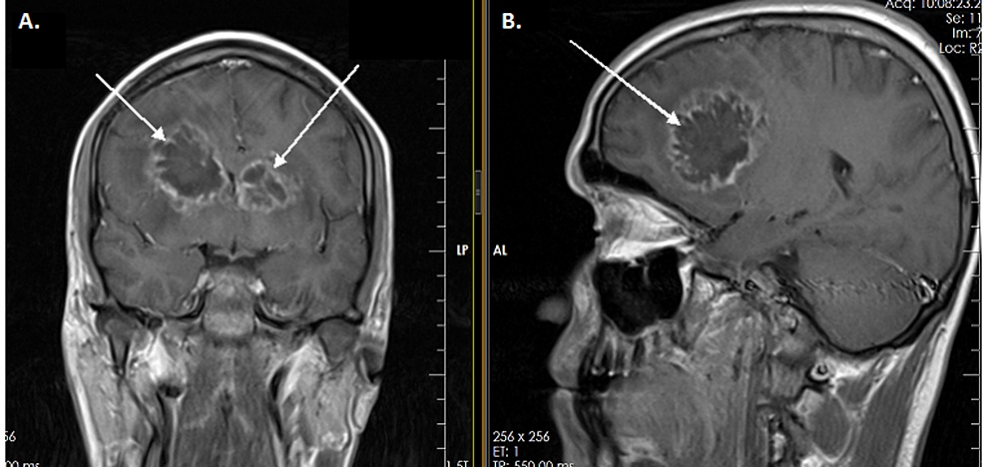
Magnetic resonance images of the brain (without contrast) showing arrows pointing to multifocal, lobulated, ring-enhancing lesions in both cerebral hemispheres surrounded with vasogenic edema (A, B)
